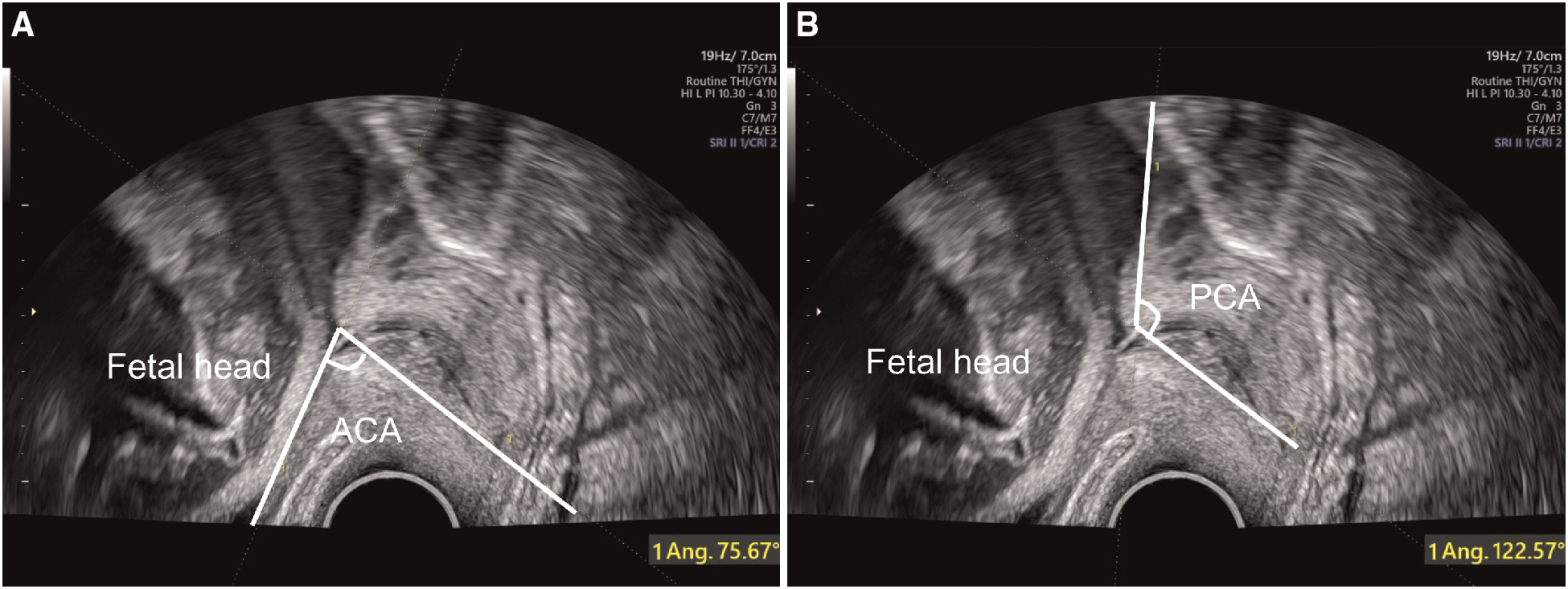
Antepartum ultrasound assessment with TVS for delivery prediction. ACA (A) and PCA (B) are assessed at the junction of the line measuring the cervical length and anterior or posterior uterine wall. TVS, transvaginal ultrasonography; ACA, anterior cervical angle; PCA, posterior cervical angle.
